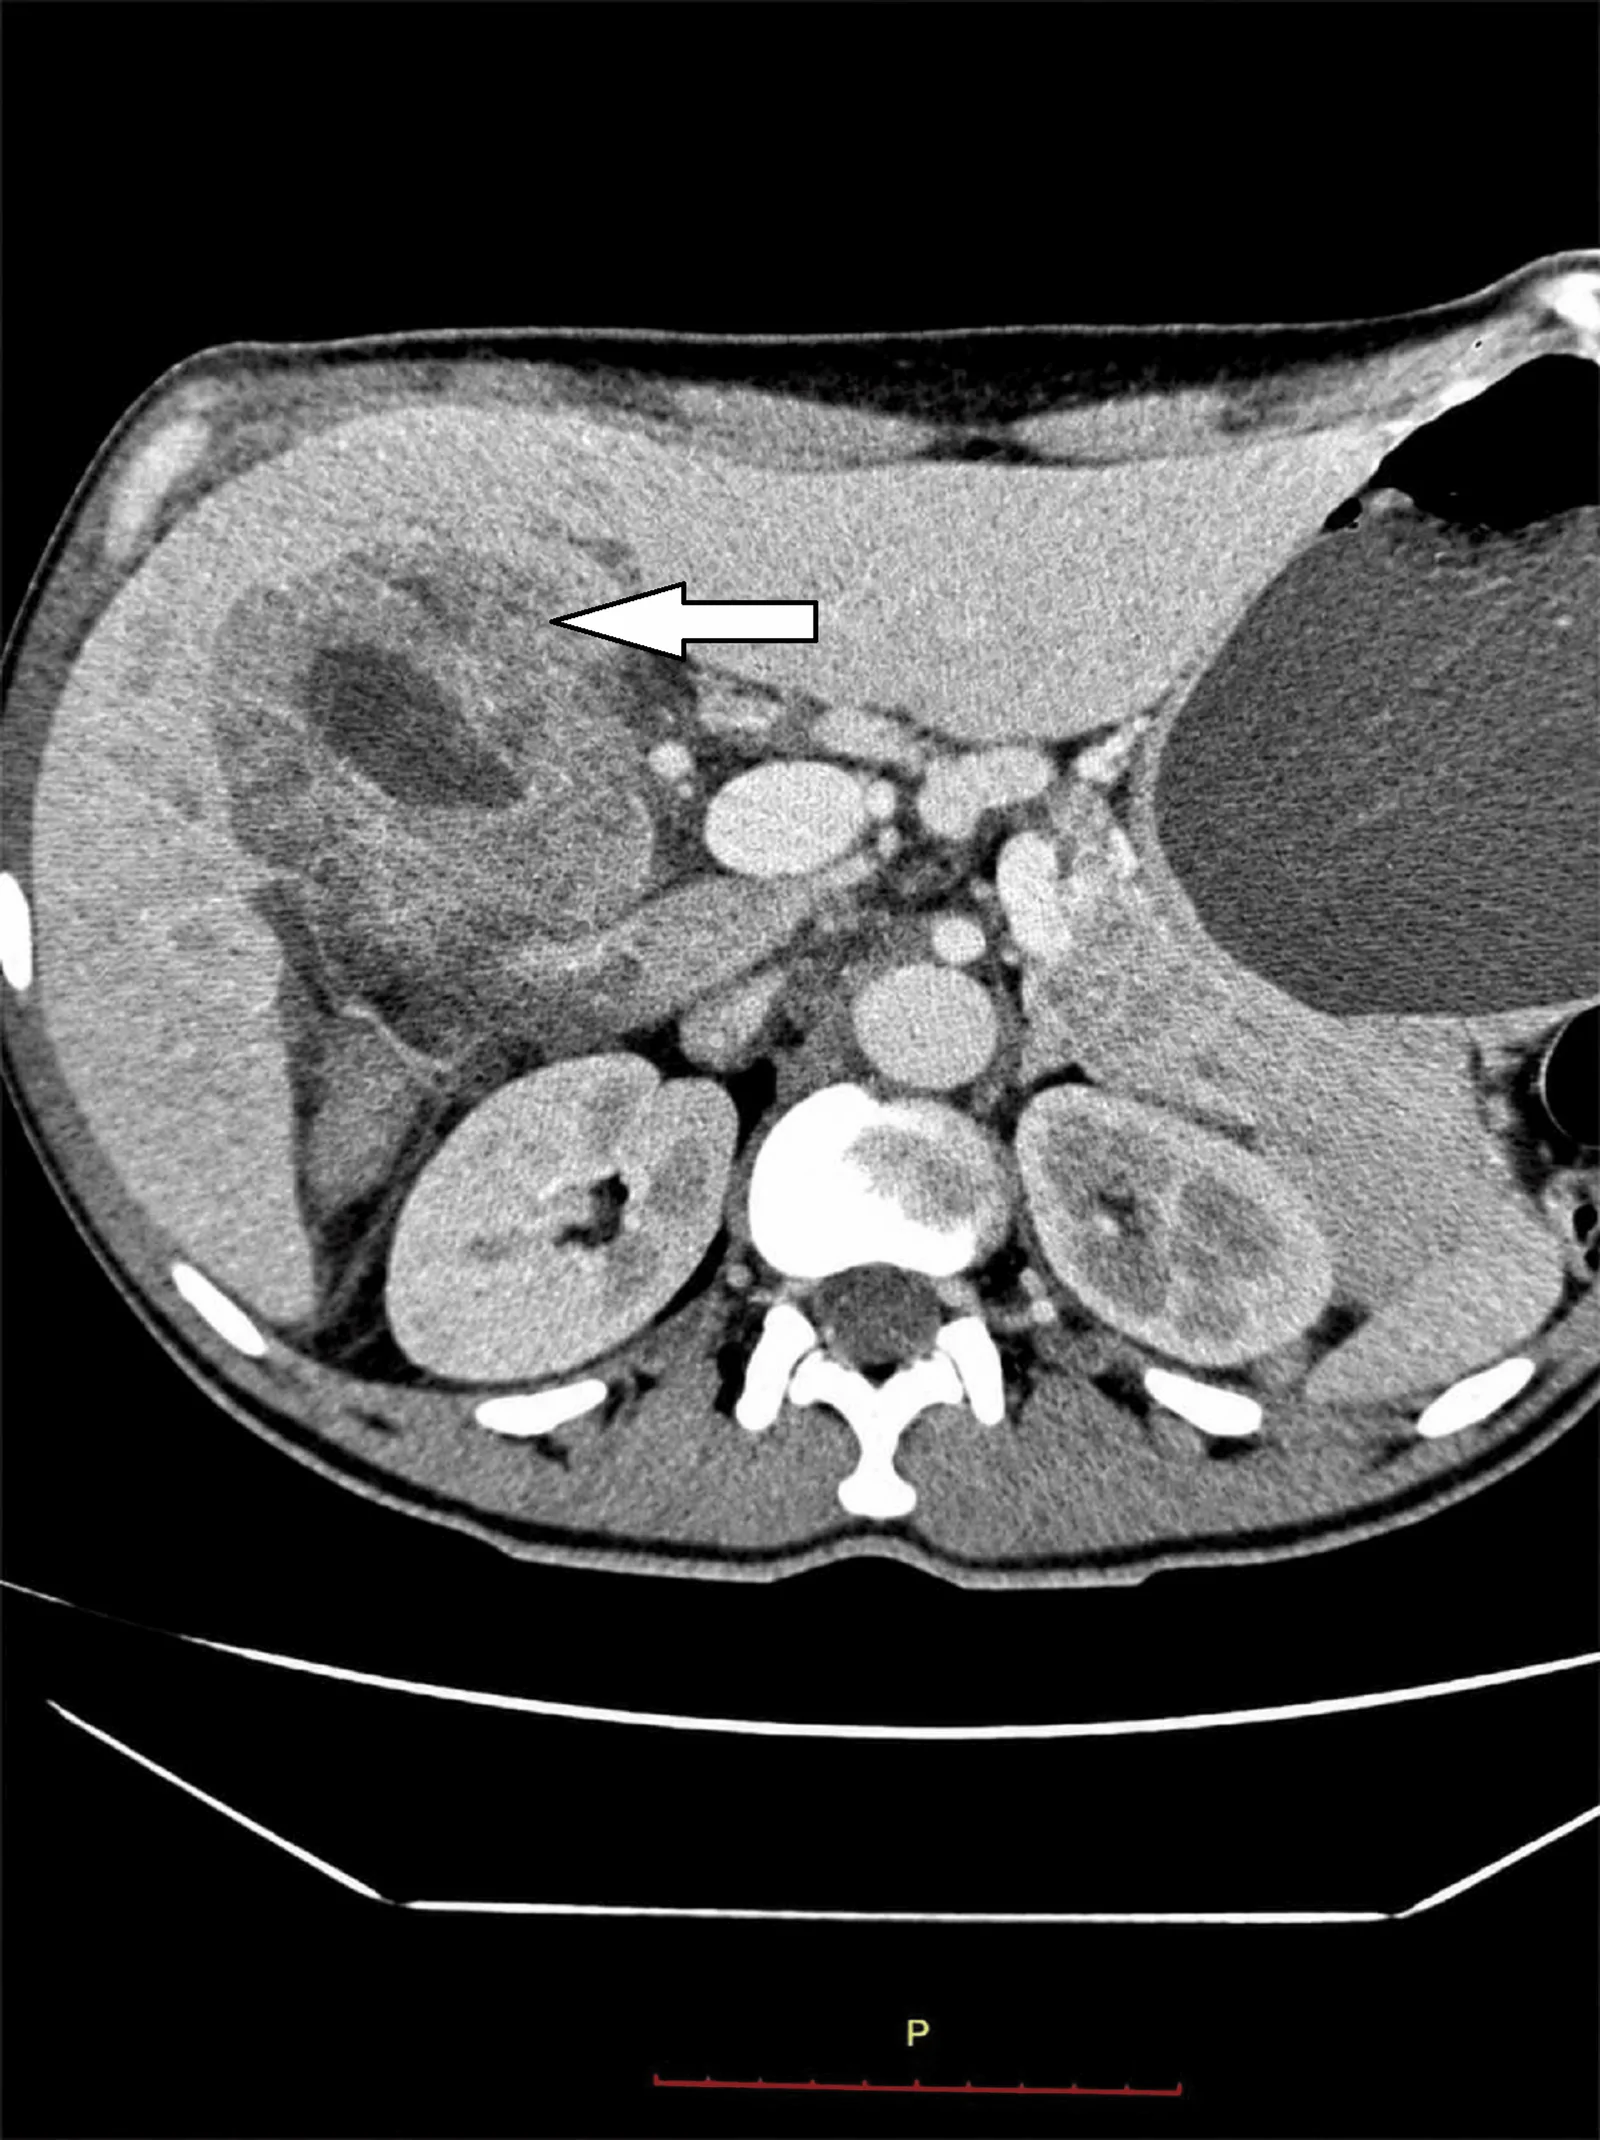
Axial contrast-enhanced computed tomography scan of the abdomen The arrow highlights a large, heterogeneous, contrast-enhancing mass originating from the gallbladder fossa with direct contiguous extension into both the hepatic parenchyma and the adjacent colon.
